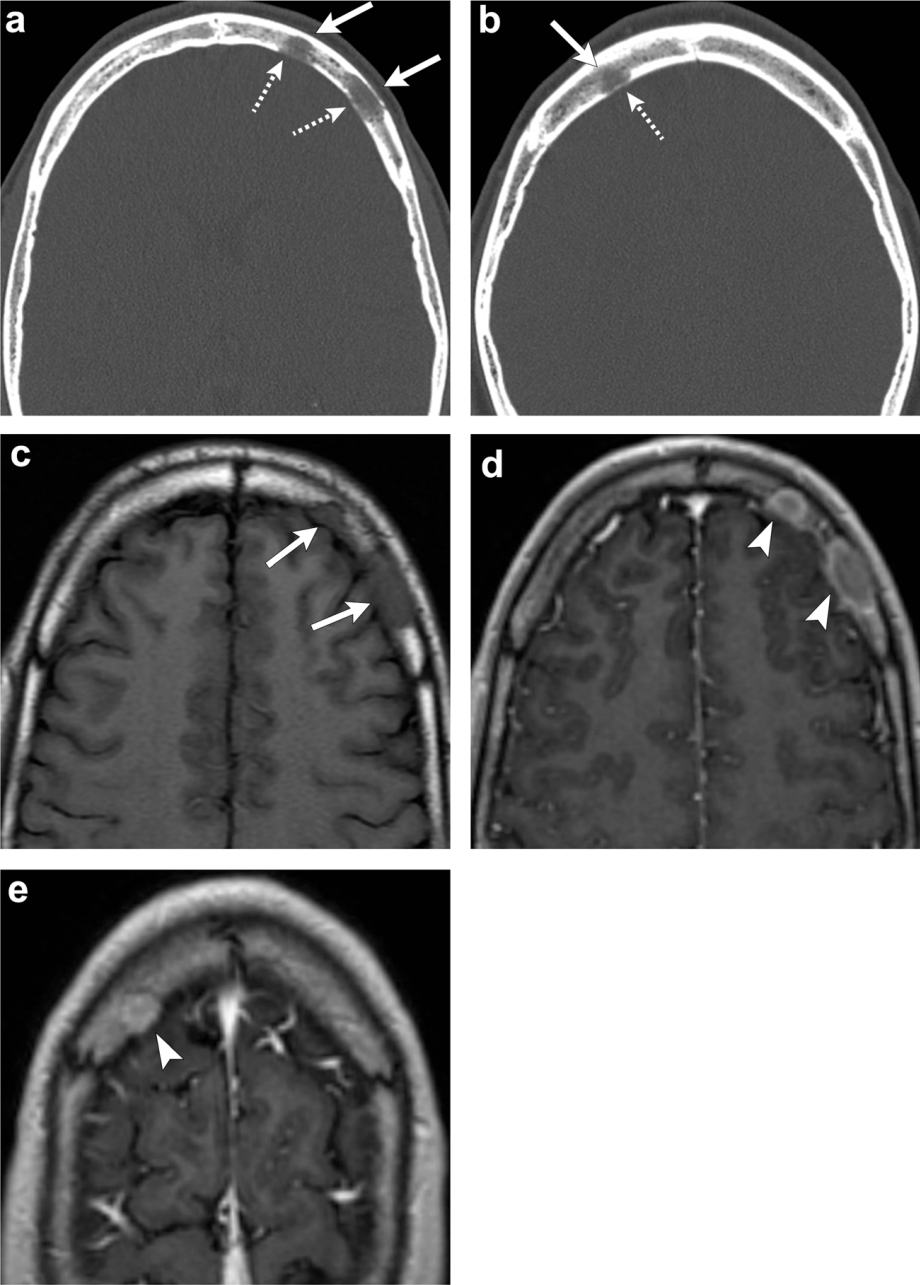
Calvaria sarcoidosis. Axial head CT (a, b) images show three lytic lesions in the diploic space of the frontal bones (*arrows*) with involvement and thinning of the cortex of the inner tables (*dashed arrows*). Axial T1-weighted imaging (c) depicts hypointense lesions (*arrows*). Post-contrast T1-weighted (d, e) images demonstrate enhancement of these lesions (*arrowheads*)
